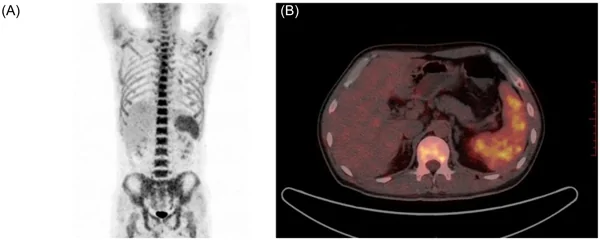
Representative PET/CT images of HNL‐HLH. (A) The lymph nodes, spleen, and bone marrow showed significantly increased FDG uptake (Case 2). (B) The mean standard uptake value of the liver was 1.3 and the spleen had a maximum standard uptake value of 4.5.
